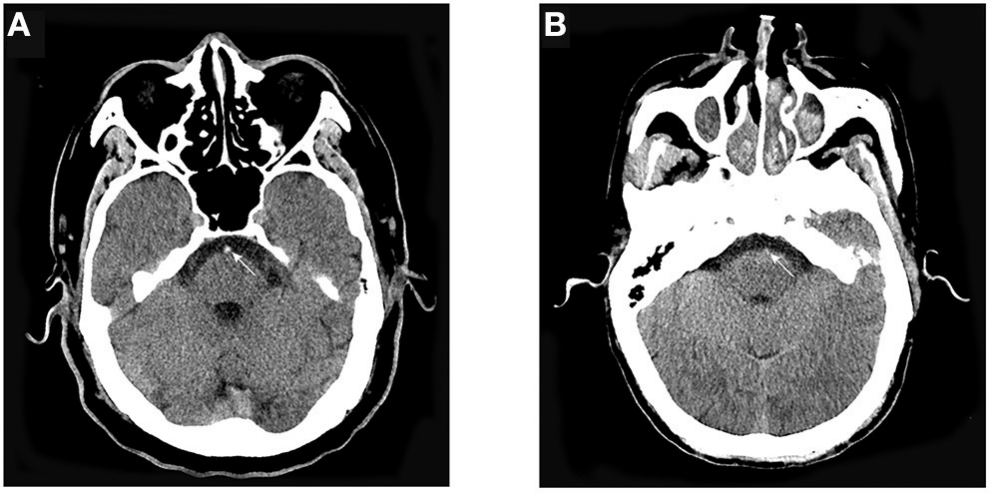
Representative cases. (A) A 62-year-old man presented acute onset of slurred speech and progressed to loss of consciousness. The basilar artery appears hyperdense on nonenhanced CT. (B) A 71-year-old man presented acute onset of dizziness and slurred speech. The basilar artery did not appear hyperdense on nonenhanced CT.
